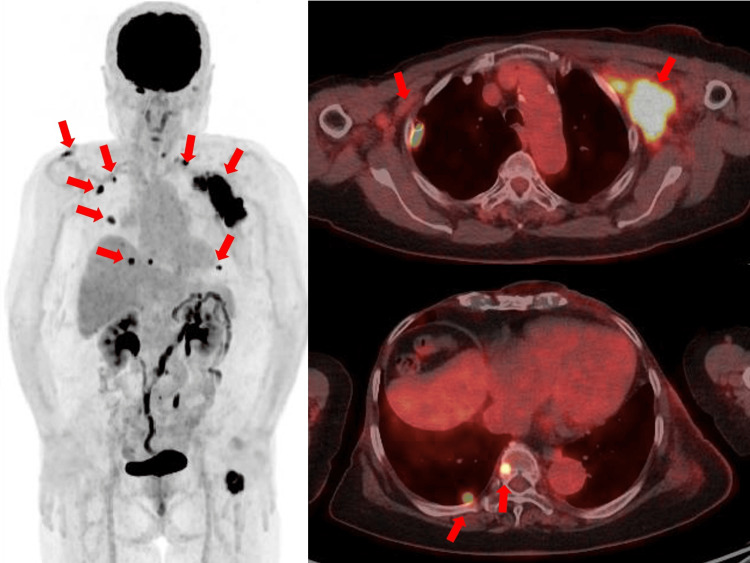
PET-CT imaging. PET-CT imaging shows recurrence in the left axilla, multiple lymph nodes, lung, and bone (arrows).
